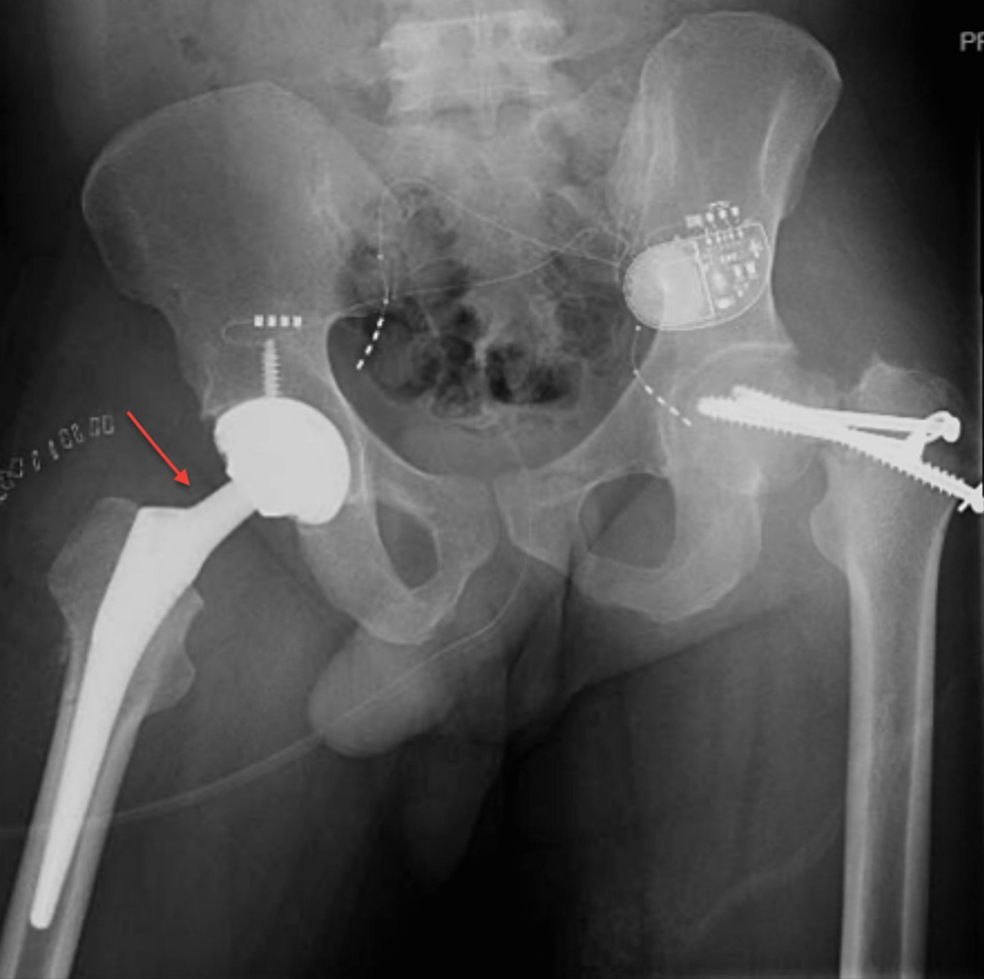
Postoperative right side total hip arthroplasty x-ray
